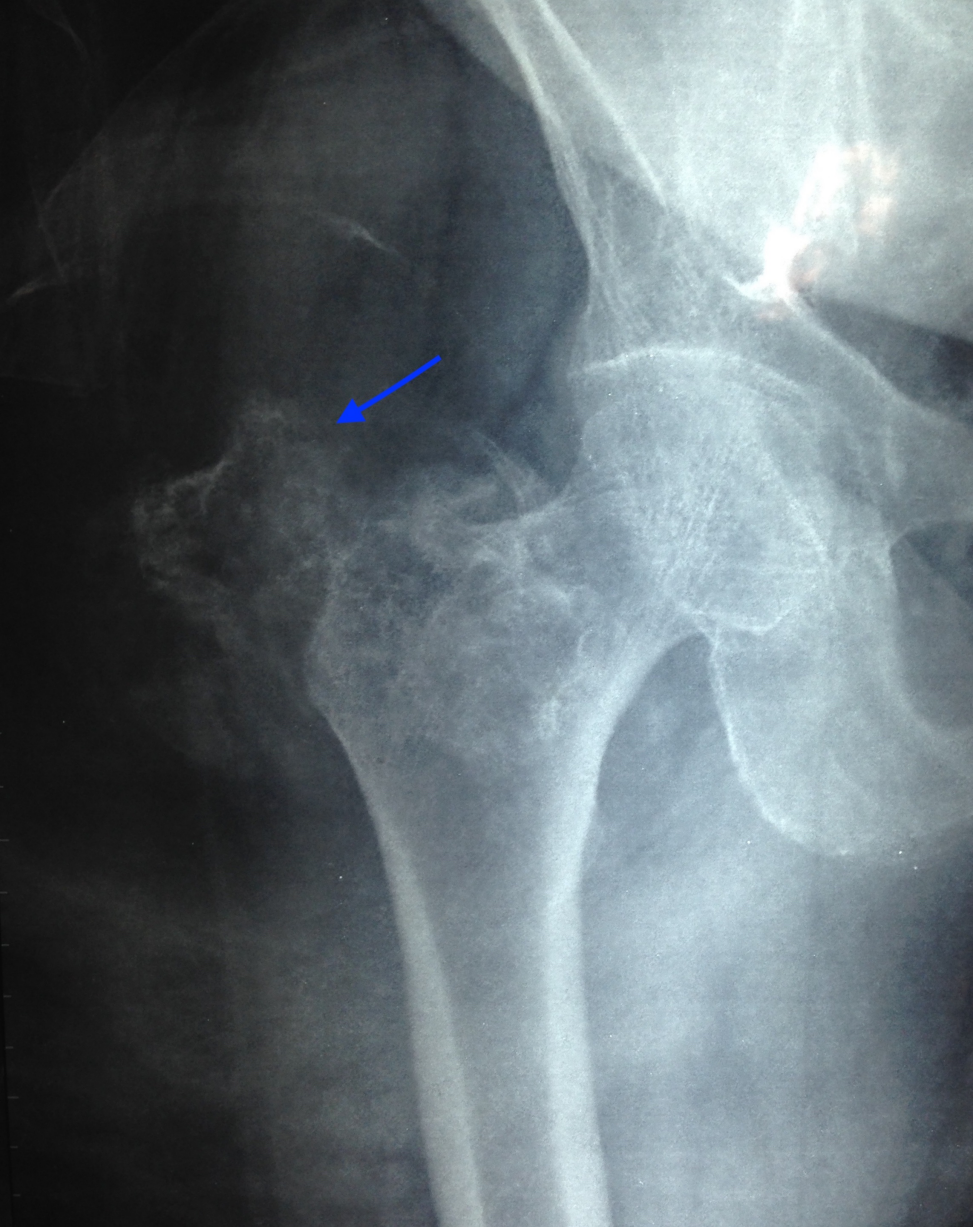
A plain X-ray showing dispersed calcification close to the periosteum of the greater trochanter of the femur
